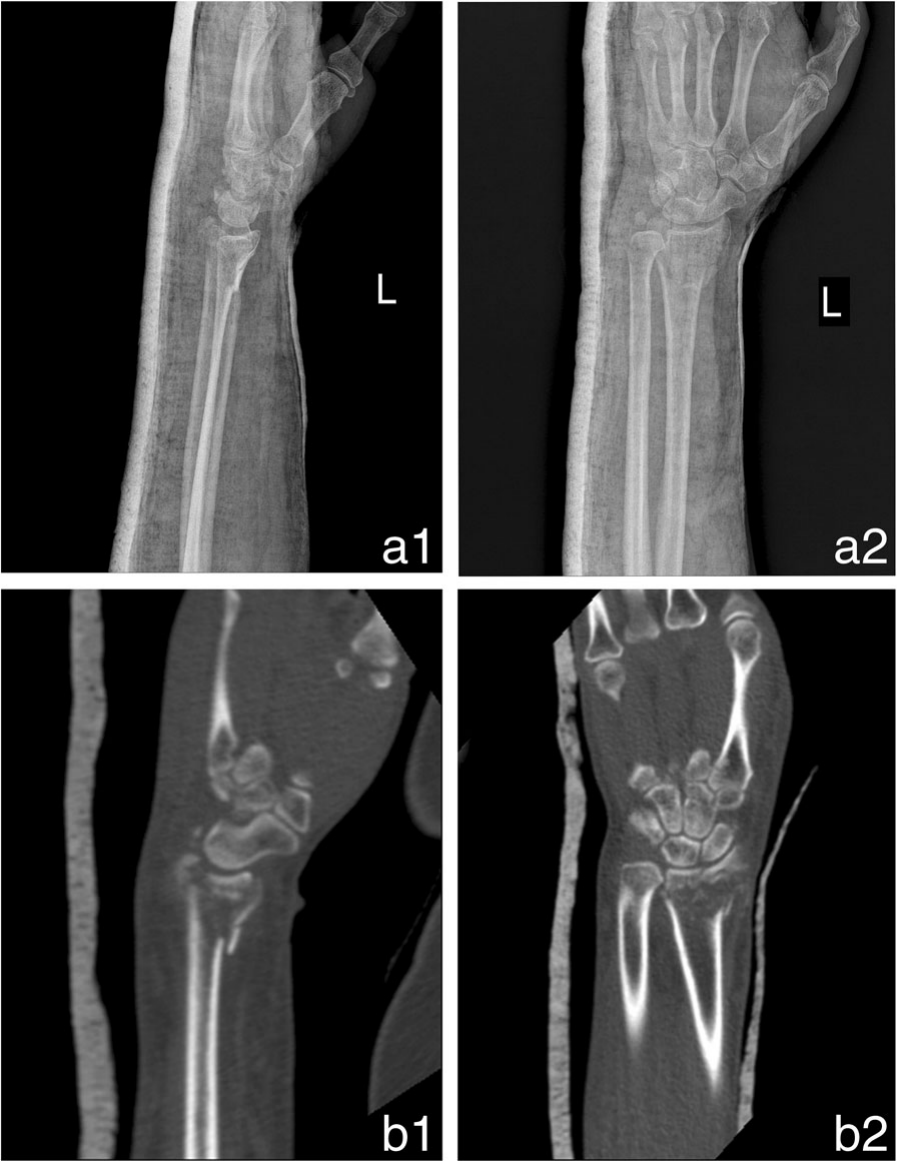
A 41-year-old man patient had a distal radius fracture, classified as AO type C1, selected as a typical case. Preoperative radiological characteristics of a distal radius fracture. a1, a2: Anteroposterior and lateral X-ray. b1, b2: Computed tomography (CT) images of the fracture
